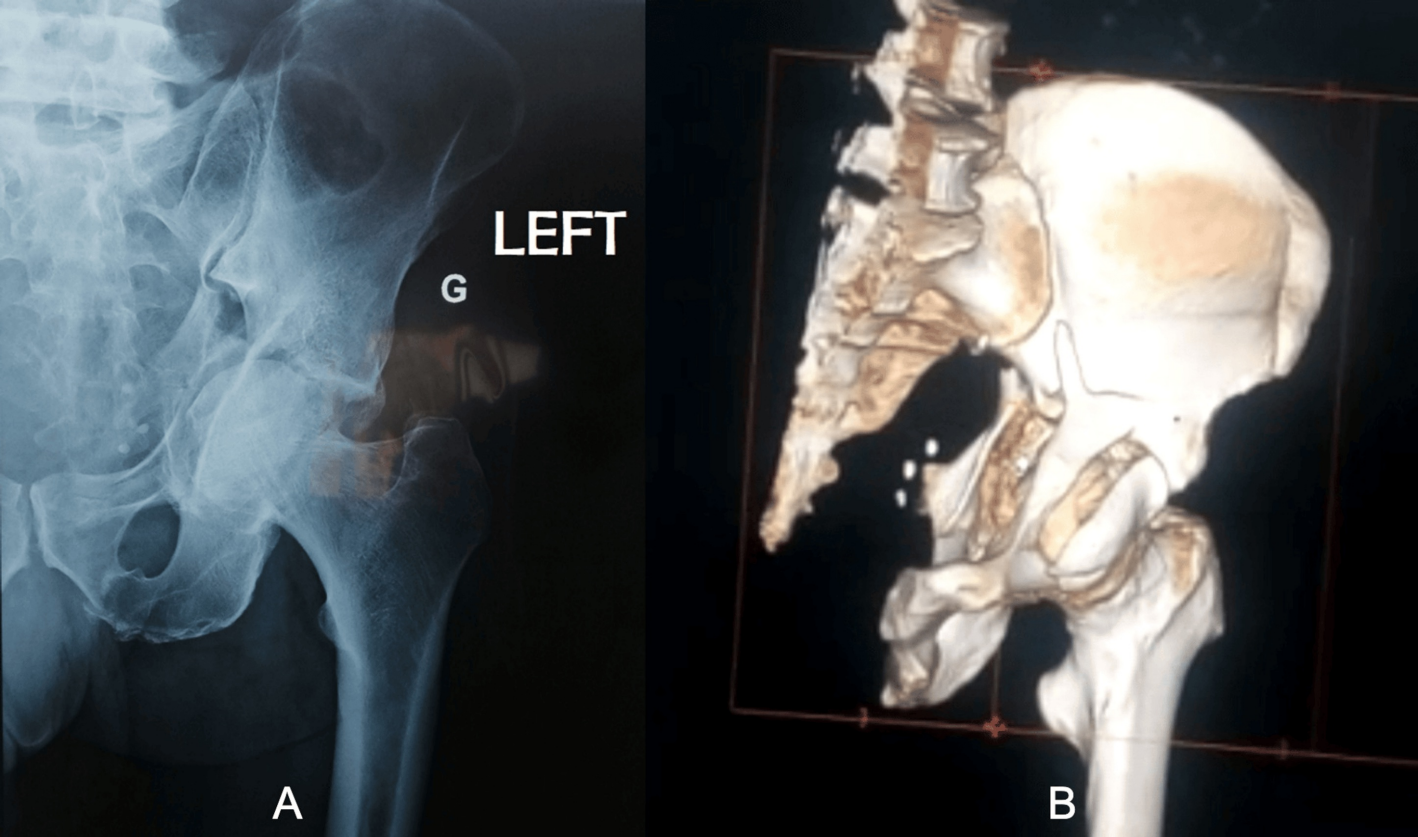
Left acetabular comminuted fracture (anterior column with posterior transverse fracture) (A) Anteroposterior view X-ray of the left hip. (B) 3D CT reconstruction of the fracture
